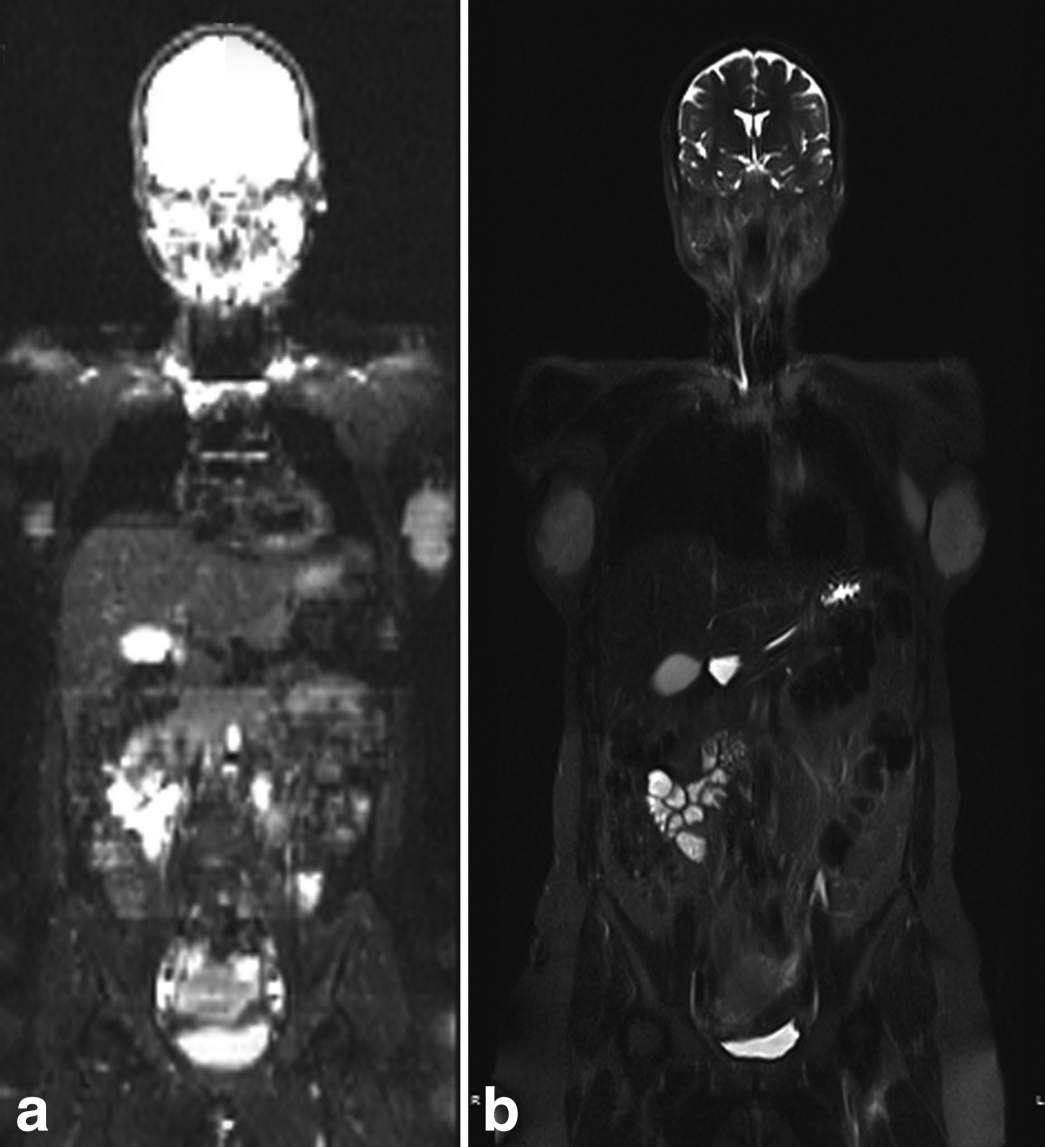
Coronal whole-body images. The b-value image at b-0, shown as white on black, represents a fat saturated fluid sensitive sequence (a), however, the purpose of DWI as a functional technique is to maximize lesion conspicuity by maximizing signal to noise. Typically this is performed with 3 × 3 mm by 5 mm thick slices, as compared with the 1.6 mm × 1.6 mm inplane STIR sequence which demonstrates exquisite anatomic detail (b).
